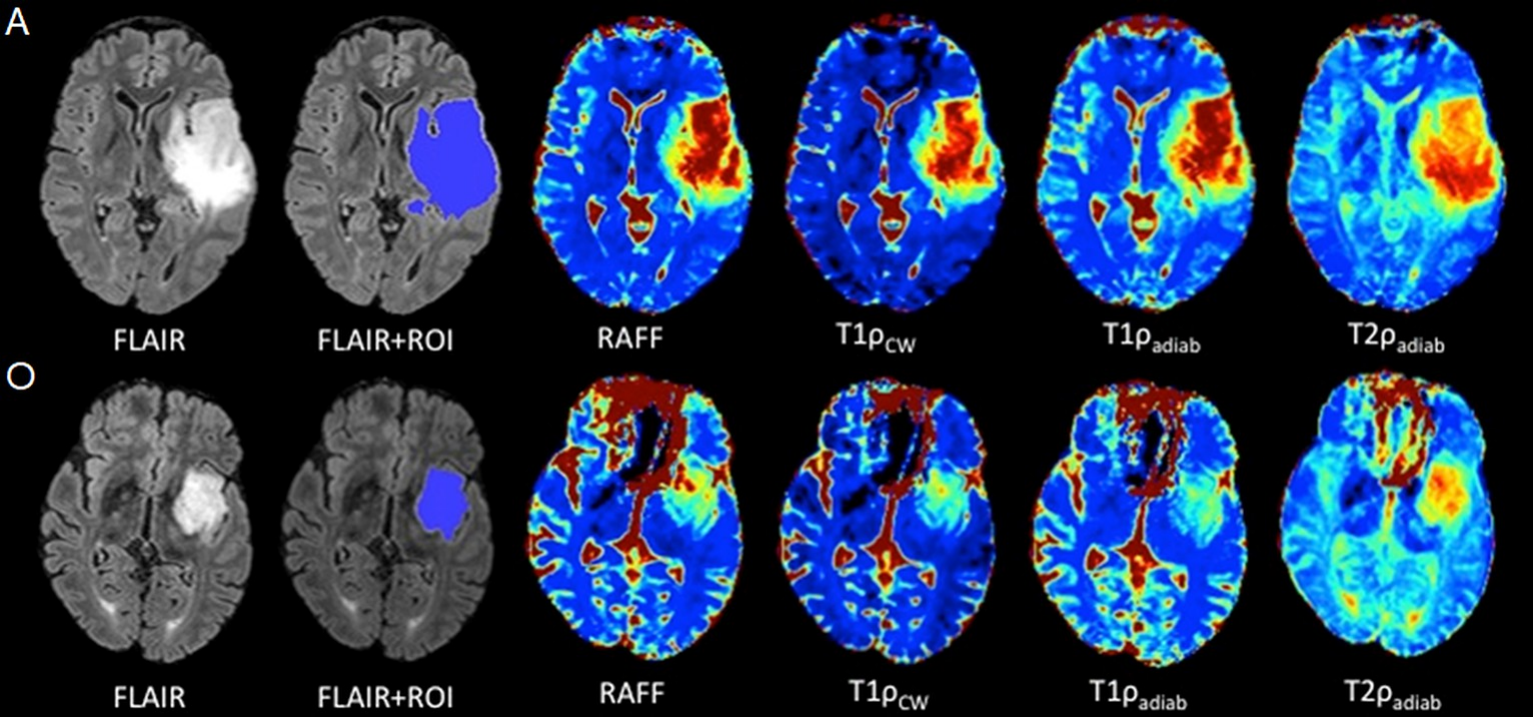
Two representative cases: 1: Diffuse astrocytoma IDH-mutant, grade 2 (A), (upper row) and 2: oligodendroglioma, 1p/19q codeleted, grade 2 (O) (bottom row) demonstrate automatic FLAIR lesion segmentation and imaging contrasts for T_RAFF2_, T_1ρcw_, T_1ρadiab_, and T_2ρadiab_ parametric maps. Same scaling was applied within the same sequence to allow comparison between the gliomas. Diffuse astrocytoma, IDH-mutant shows elevated values in all parametric maps compared to oligodendroglioma, IDH-mutant and 1p/19q codeleted. This trend was observed across the cohort, however, without statistical significance.
